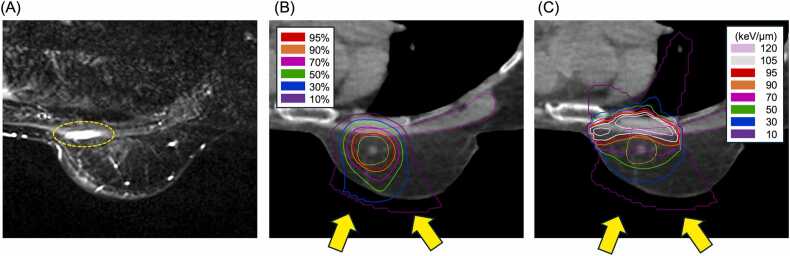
A pectoral myositis and dose distributions of C-ion RT. (A) A representative MRI of pectoral myositis after 3 months of C-ion RT. (B) RBE-weighted dose distribution of C-ion RT on the CT image for the same patient. Isodose lines indicate percentages of the prescribed dose (50 Gy [RBE]). (C) LETd distribution of C-ion RT on the CT image for the same patient. Isodose lines indicate the values of LETd. Pectoral myositis was determined based on changes in a contrast-enhanced MRI of the irradiated area, as indicated by the yellow dotted line (A). The pectoralis major muscle is surrounded by a magenta line. Yellow arrows indicate the direction of the C-ion RT (B) and (C). Abbreviations: C-ion RT, carbon-ion radiation therapy; CT, computed tomography; LETd, dose-averaged linear energy transfer; MRI, magnetic resonance imaging; and RBE, relative biological effectiveness.
